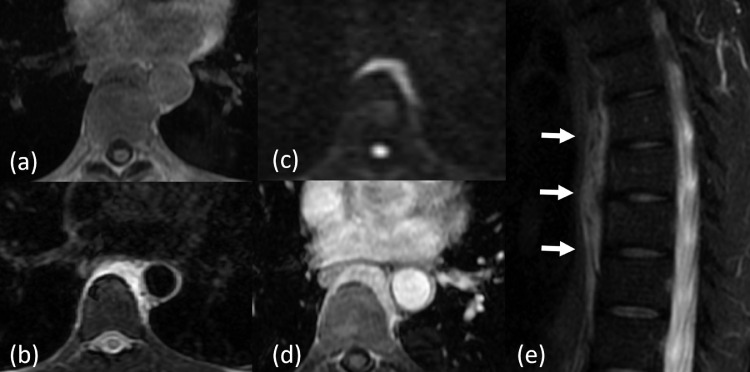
Magnetic resonance images of the thoracic spine. (a) The mass shows low signal intensity of the T1-weighed gradient-echo image. (b) Fat suppressed T2-weighted image revealing high signal intensity. (c) Diffusion-weighted image showing diffusion limitation. (d and e) Gadolinium-enhanced dynamic magnetic resonance imaging reveals the overall delayed contrast enhancement of the mass (arrows).
